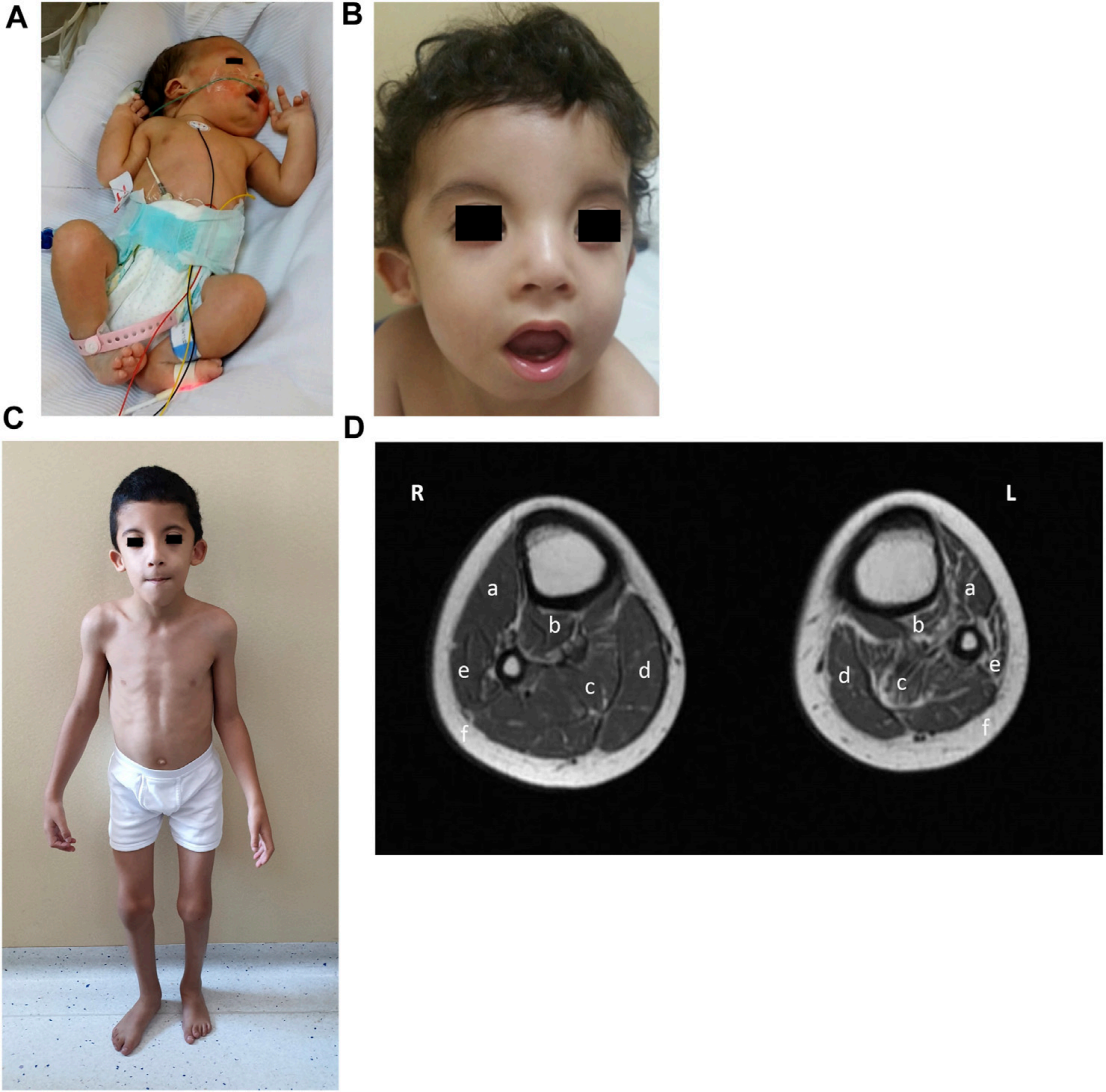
Phenotype of patient 1. (A) Clinical phenotype at the age of 2 days: bilateral generalized arthrogryposis with predominant bilateral elbow and knee flexion contractures, right foot talipes equinovarus, and left foot vertical talipes. (B) Clinical phenotype at the age of 18 months: wide forehead, bitemporal narrowing, hypertelorism, bilateral epicanthus, divergent strabismus, small depressed nasal bridge, small nose, short columella, anteverted nares, smooth and long filtrum, micrognathia, myopathic face appearance with facial muscle weakness, wide and opened mouth with an everted lower lip, high arched palate, small and anterior rotated ears with smooth helix and antihelix, and anterior and uplifted ear lobules. (C) Clinical phenotype at the age of 7 years and 10 months: myopathic facies with the same previous particularities, high-arched palate, overcrowded teeth, abnormal dental eruption, generalized thin build, hyperlordotic spine, mild elbow and knee contraction bilateral with hypotrophy of calf muscles, more marked on the left side, and overlapping second and third toes bilaterally. (D) MRI lower-limb (plain) (R-right, L-left): T1 axial of the calves showing mild atrophy of right gastrocnemius (c) and right soleus (d) muscles and subtle fatty infiltration; moderate atrophy of left anterior tibialis (a), posterior tibialis (b), gastrocnemius (c), and peroneal (e) muscles with prominent fatty infiltration; with a relative increase of the subcutaneous fat thickness in the left calf compared to the right one.
